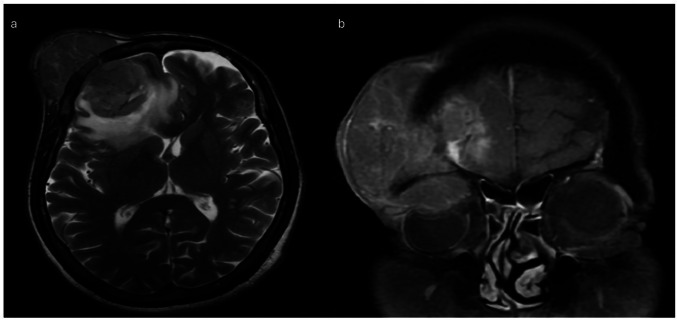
a Magnetic resonance imaging with T2 sequence depicting right frontal heterogeneously hyperintense partially hemorrhagic lesion arising from the right frontal bone with associated soft tissue component and intracranial extradural extension. Intraorbital component with mass effect compressing the right frontal lobe with moderate perilesional edema and midline shift is also noted. b MRI head T2 coronal view depicting frontal bone lytic lesion with intraorbital and intraconal extension. It shows loss of fat planes with the superior rectus muscle and is abutting the superior aspect of the globe. Other features seen include bone lysis, loss of bone marrow signal, and asymmetry in diploic space
